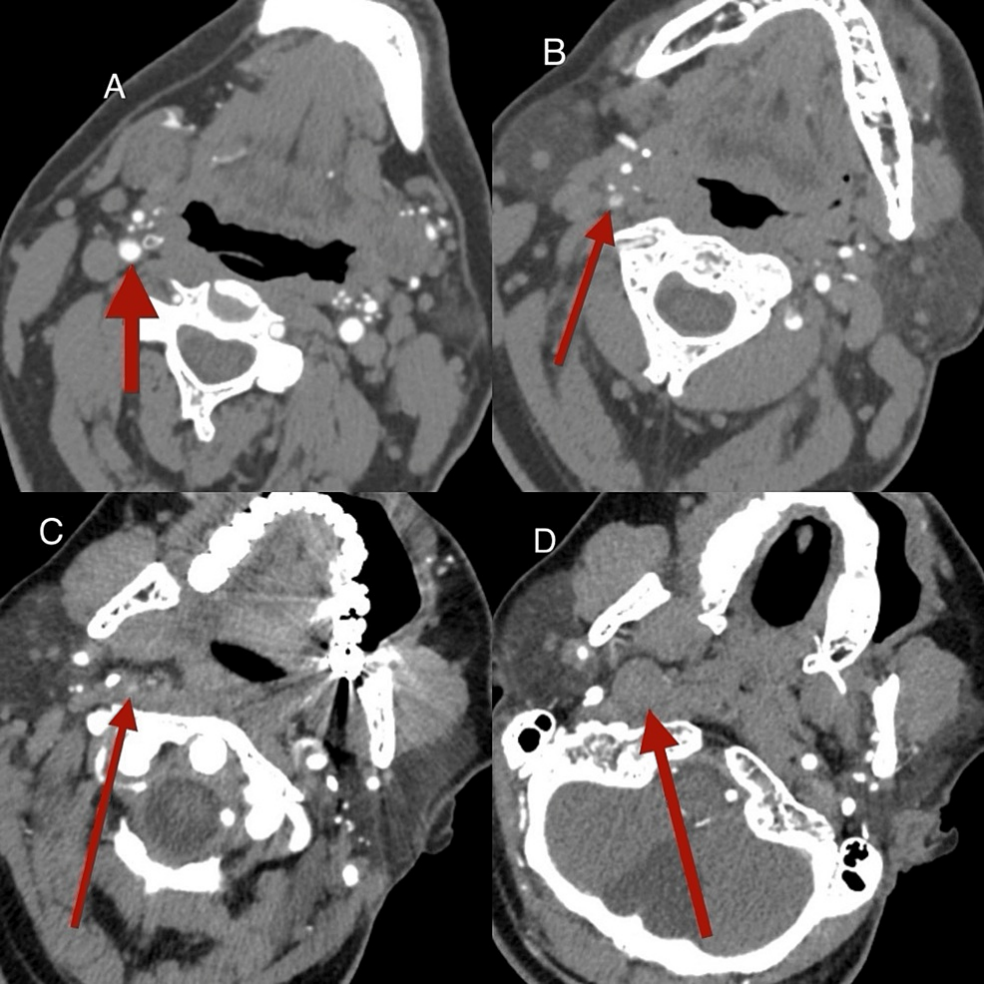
CT showing ICA dissection and thrombus (A) Normal carotid artery below the level of dissection; (B) Beginning of right ICA dissection: attenuation of the contrast product in the proximal segment and reduction of arterial lumen; (C) Right ICA dissection just medial to the large styloid process; (D) Enlarged and completely thrombosed right ICA at the skull base, characterized by absence of contrast product ICA: internal carotid artery
